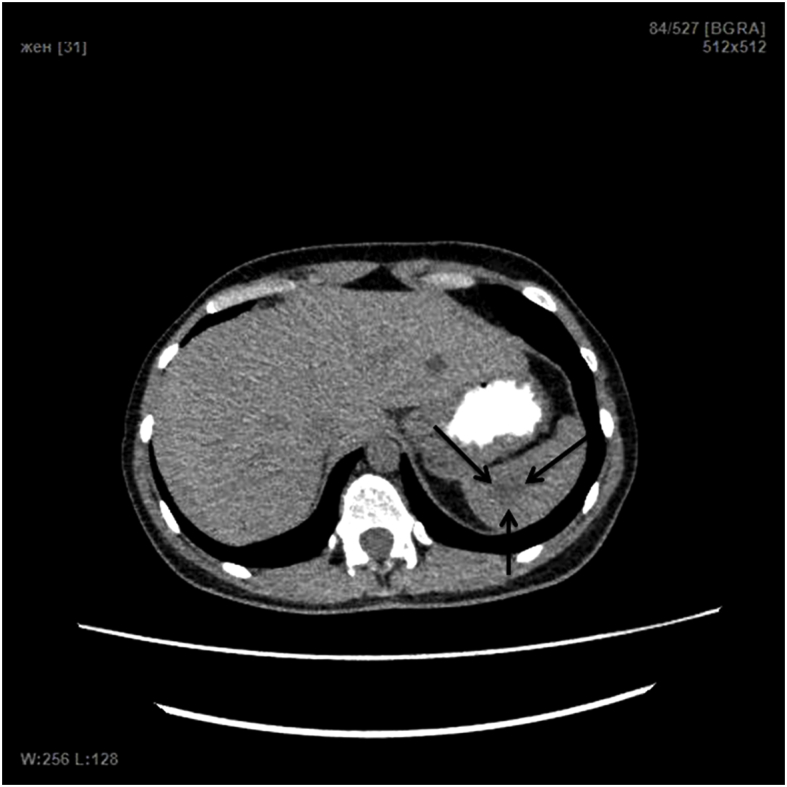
Four months post-surgery CT scan of the abdominal region on October 22, 2018. Note the residual cavity of the splenic cyst of 3 × 1.7 × 1.9 cm.
